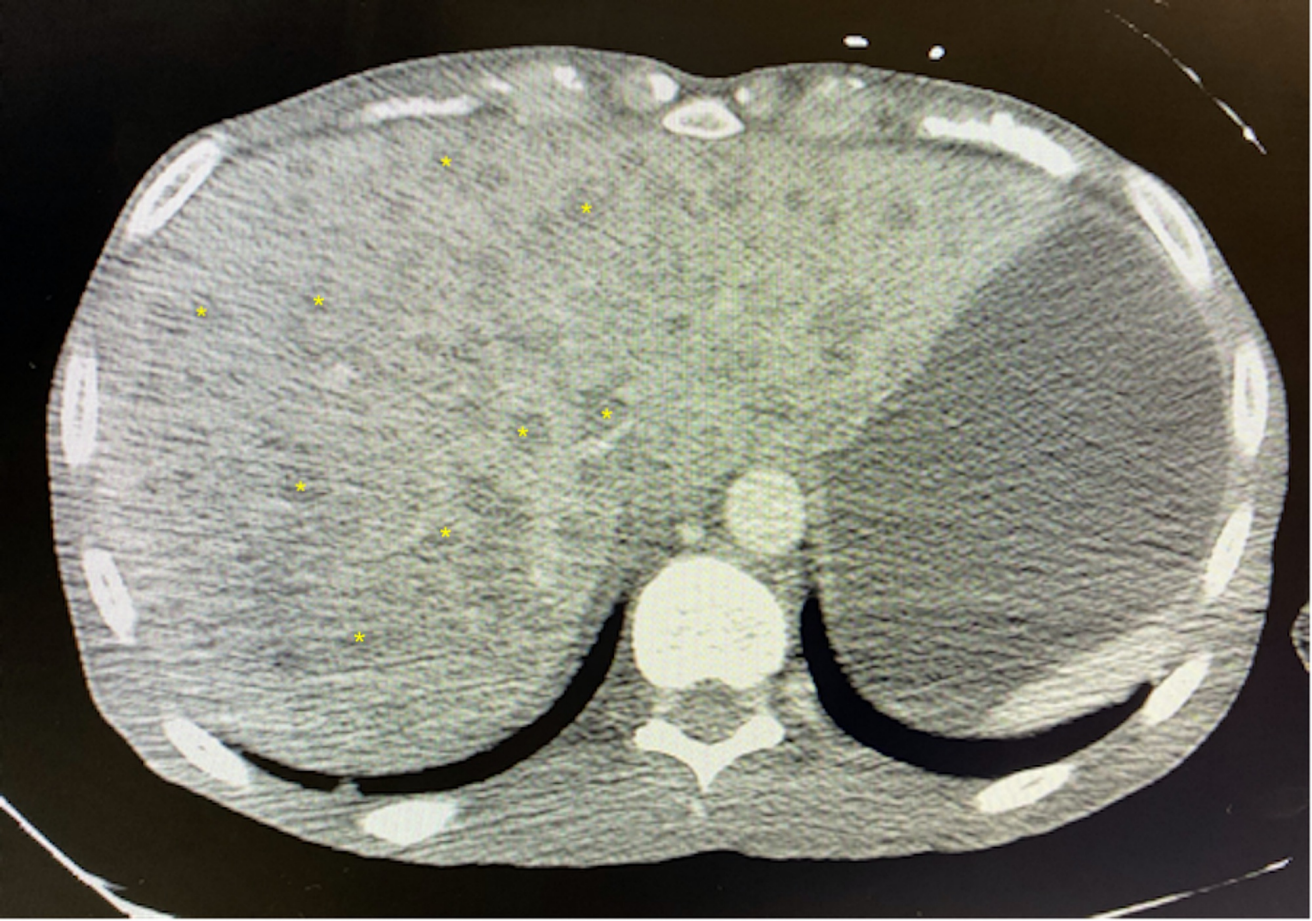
CT scan showing multiple liver nodules and masses (yellow asterisks) CT, Computed tomography.
